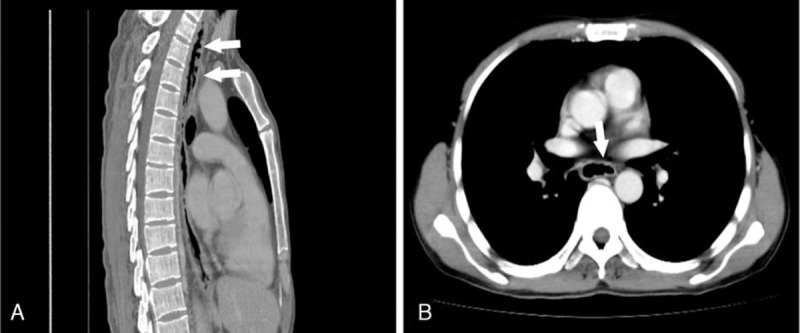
Computed tomography (CT) scan of an esophageal lesion. CT showed the esophagus wall was rigid and demonstrated rugosity (arrows) in the sagittal view (A) and transverse section (B).
